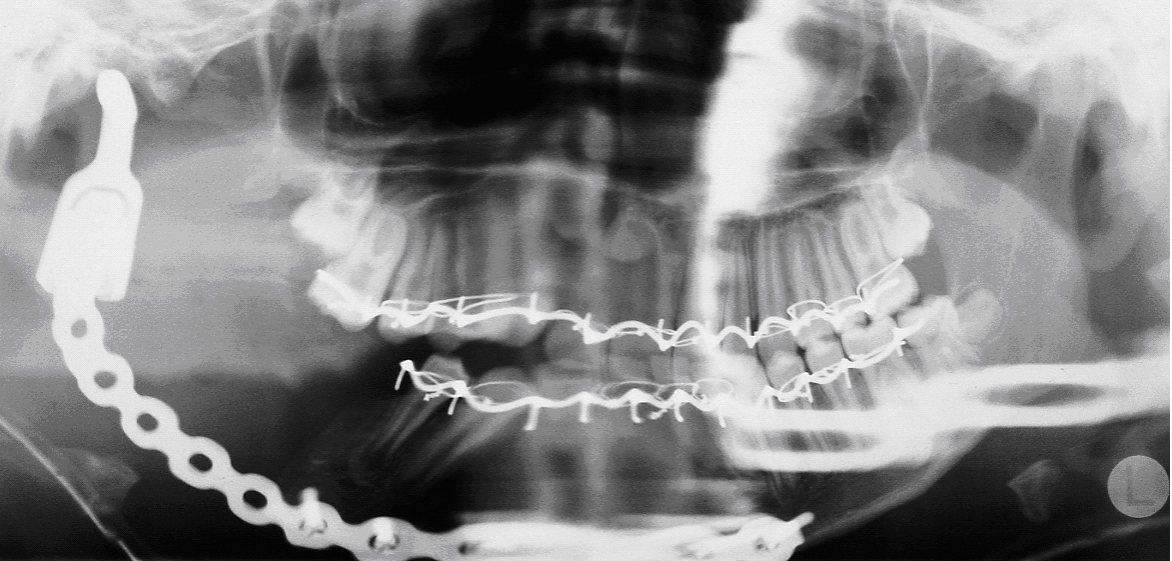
Panoramic radiography post operation. The combined Recontruction locking plate with artificial condylus can be seen.
